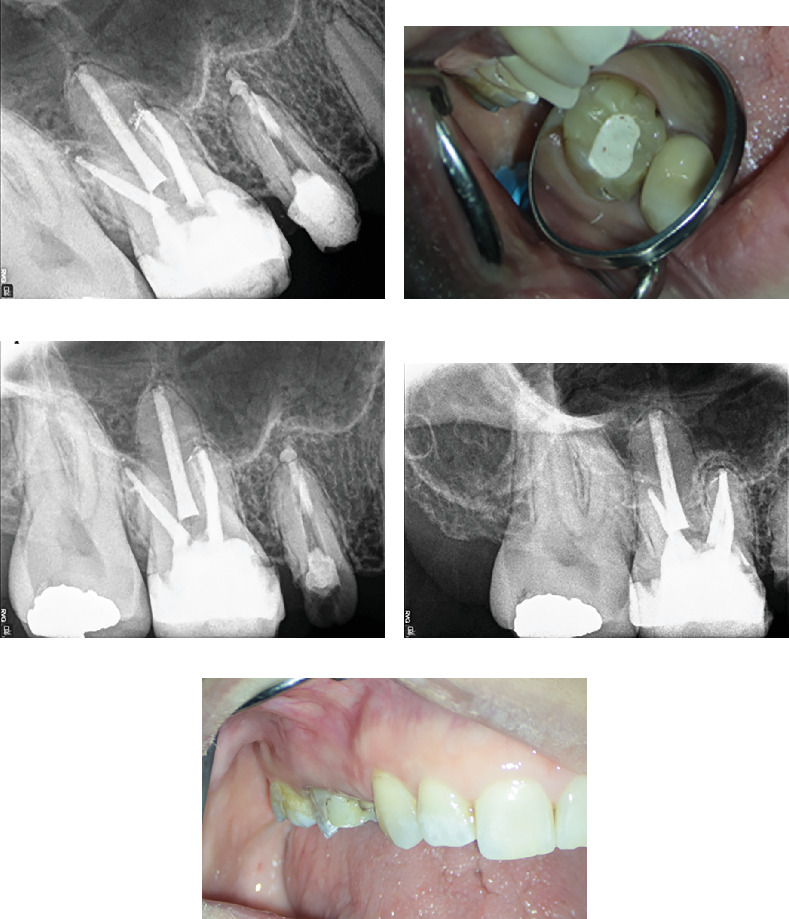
(a) Six-month radiographic follow-up shows complete resolution of the periapical radiolucency, while (b) provides intraoral photography of the treated tooth after 6 months. (c, d) Periapical radiographs at the 2-year and 3-month follow-up reveal normal periradicular structures with no signs of pathology. (e) Intraoral photograph at 2 years and 3 months posttreatment showing stable clinical conditions and absence of pathological changes.
